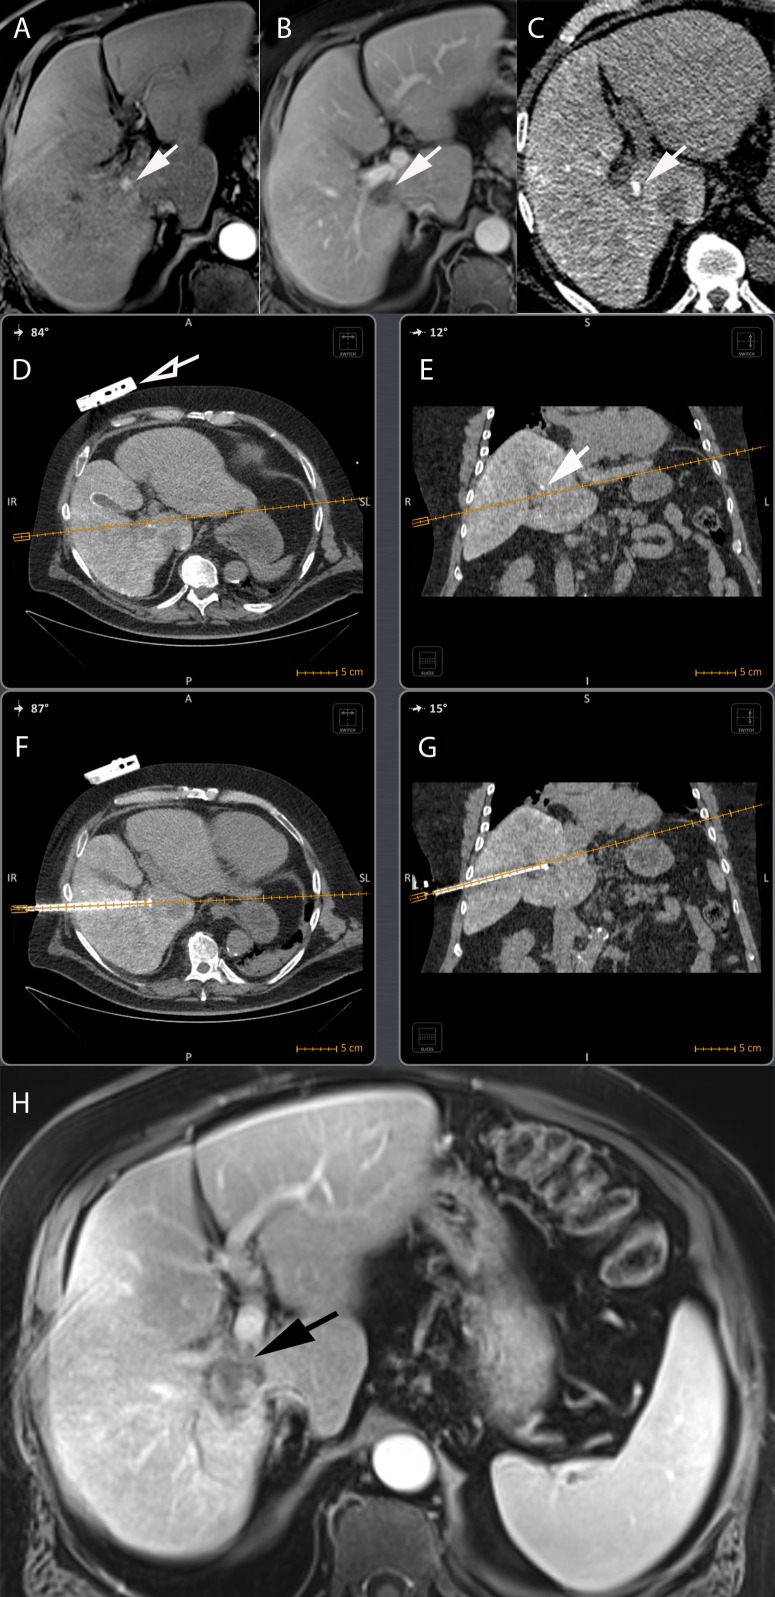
Electromagnetic CT navigation system for precise per-procedural guidance in a 67-year-old male with alcoholic cirrhosis and recurrent HCC following RFA. (A) Axial contrast-enhanced (arterial phase) T1-weighted MR image showing nodular enhancement adjacent to an ablation scare consistent with HCC recurrence following RFA (arrow). (B) Axial contrast-enhanced (portal venous phase) T1-weighted MR image showing tumor washout of the lesion, typical hallmark of HCC (arrow). (C) The lesion was neither visible on ultrasound nor unenhanced/contrast-enhanced CT. An intra-arterial hepatic administration of Lipiodol was thus performed to “tag” the tumor which became visible on unenhanced CT (arrow). The navigation system displays the needle path in real time in any plane wanted, here on axial (D) and coronal (E) views of a CT fused with the electromagnetic CT navigation system (Imactis SAS, La Tronche, France). The planned needle tract can thus be seen before the RFA probe is inserted in the patient. The fiducial of the navigation system is visible on the patient skin in (D) (hollow arrow). The tumor containing the Lipiodol is visible in (E) (arrow). Axial (F) and coronal (G) oblique views showing out-of-plane needle placement directly into the target lesion avoiding critical structures such as right portal vein and hepatic duct. (H) Axial contrast-enhanced (arterial phase) T1-weighted MR image showing complete ablation with no residual suspect area of enhancement (black arrow).
